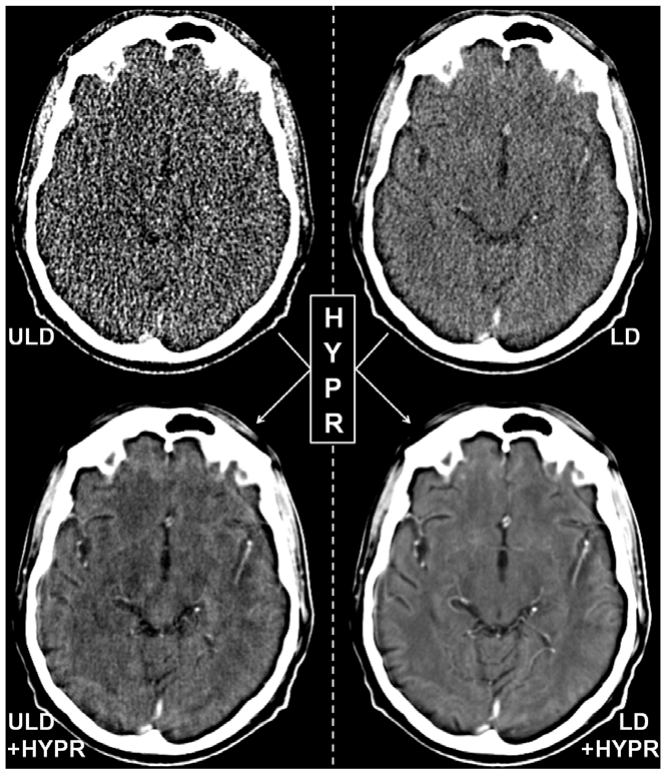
Example images of ultra low dose (ULD), HYPR-LR-post-processed ultra low dose (ULD+HYPR), low dose (LD) and HYPR-LR-post-processed low dose (HYPR+LD) brain perfusion CT of a 58-years old male patient with no pathology. The images are all reconstructed with the same slice thickness (5 mm) and presented with identical window settings.
